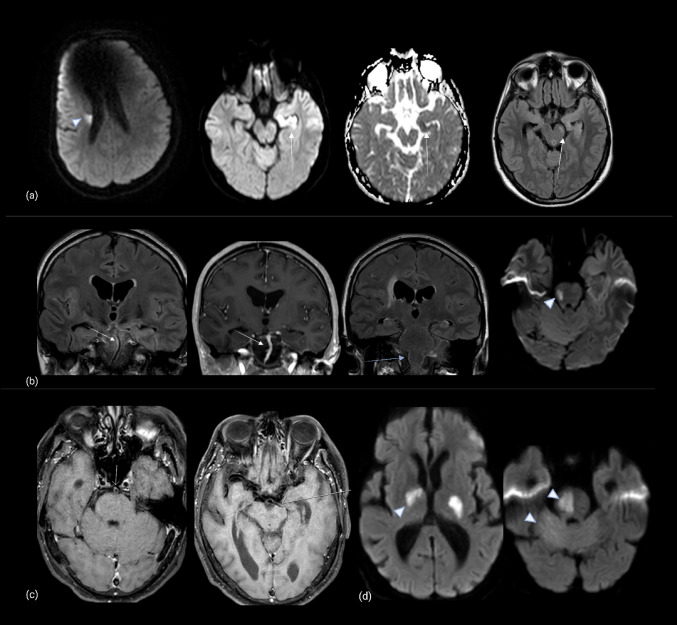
MRI of the cerebrum 2012–2023, case 2 and 3. a (Case 2): acute infarction in the right centrum semiovale on DWI (white arrowhead) (USA 2012), which grew in size on control MRI 12 days later (not shown, DK 2012), and acute infarction in the left temporal lobe on DWI, ADC and FLAIR (white arrows) (DK 2017), respectively. b Longitudinal (peri)vascular enhancement of the BA on post-contrast coronal FLAIR (long white arrow) with irregular abluminal contour on postcontrast T1 sequences (short white arrow). Appreciate the much thinner basilar lumen compared to the width of contrast enhancement. Also, leptomeningeal enhancement around the brainstem is seen (blue arrow) and cranial nerves (not shown (2017)). Acute pontine infarction on the right side on DWI (white arrowhead, 2018)). c (Case 3): HRVWI-MRI with concentric enhancement of the BA, PCA and left VA, respectively (white arrows). d Acute infarction with DWI lesions in the right thalamus, pons and cerebellum (white arrowheads). HRVWI-MRI (MRI High resolution vessel wall imaging), BA (basilar artery), PCA (posterior cerebral artery), VA (vertebral artery), BA (basilar artery), MCA (middle cerebral artery), FLAIR (fluid attenuated inversion recovery), DWI (diffusion-weighted magnetic resonance imaging), ADC (apparent diffusion coefficient), MRI (magnetic resonance imaging)
